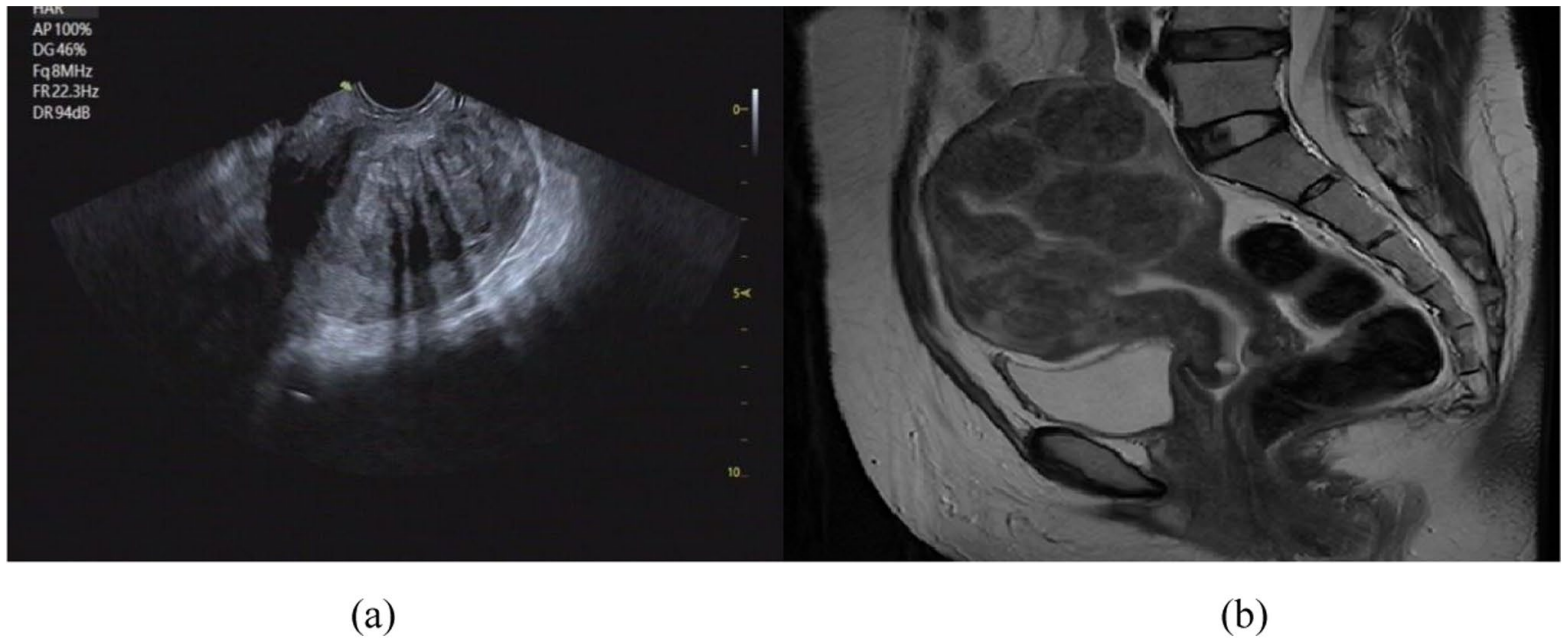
Comparison of imaging effect between US image (a) and MRI image (b). (a) US image of uterine fibroids; (b) MR image of uterine fibroids.
